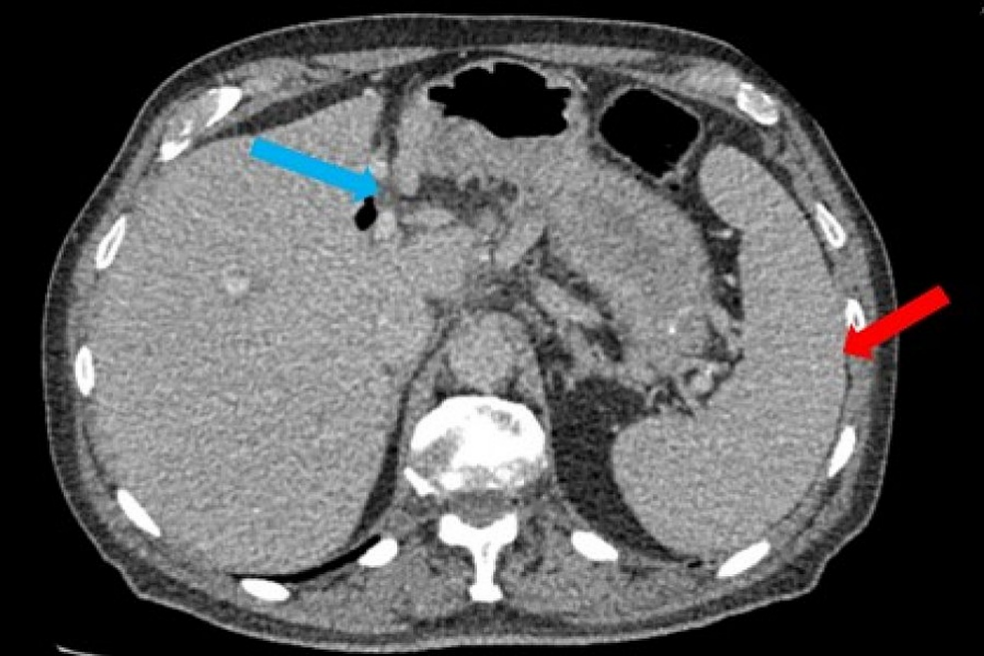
CT abdomen showing splenomegaly of 18.5 cm (red arrow) and multiple mesenteric lymph nodes (blue arrow)
